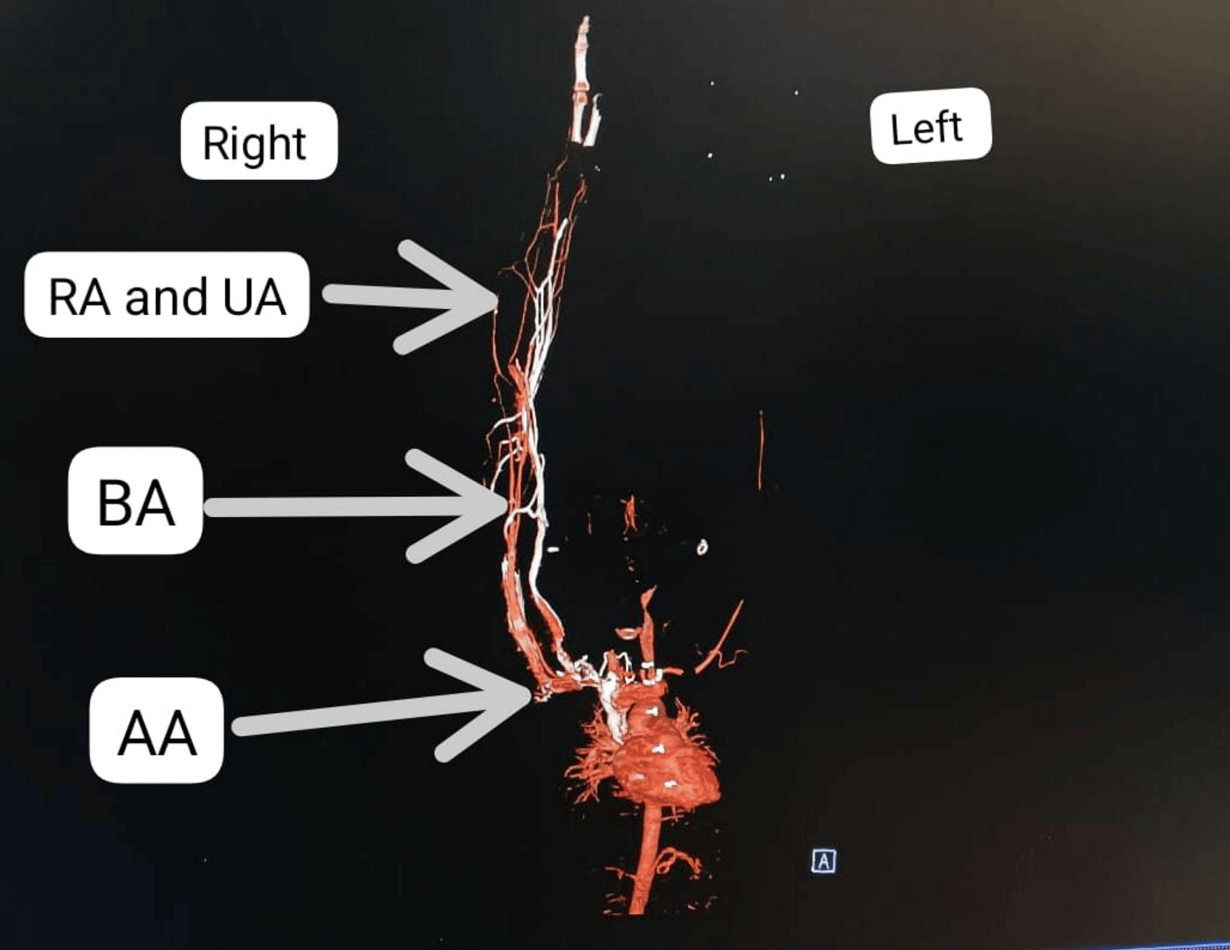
Volume-rendered CT angiographic study of the bilateral upper limbs in the coronal section, depicting near-complete thrombosis of the left subclavian and axillary arteries and complete thrombosis of the left radial and ulnar arteries. RA = radial artery; UA = ulnar artery; BA = brachial artery; AA = axillary artery
